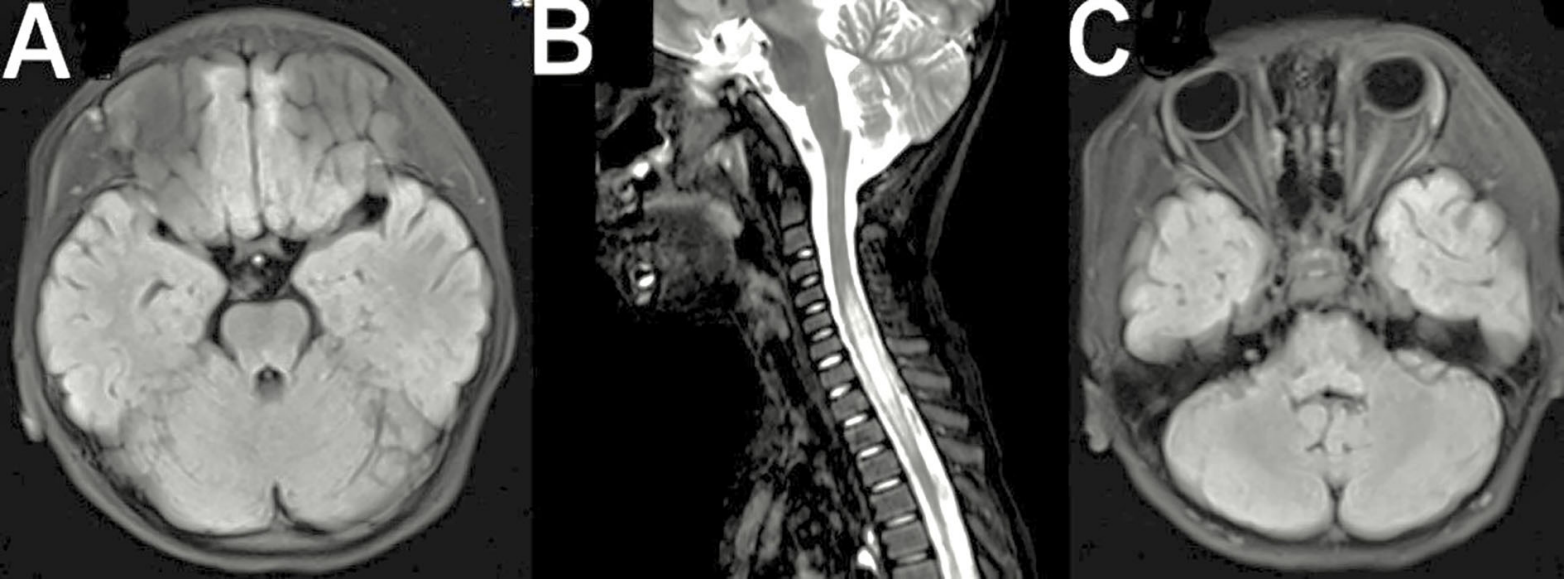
(A) Axial FLAIR brain MRI showing hyperintense lesions in the brainstem and cerebellum. (B) Sagittal T2-weighted MRI of the cervical spine demonstrating longitudinally extensive transverse myelitis (LETM). (C) Axial FLAIR MRI indicating possible optic nerve involvement.
